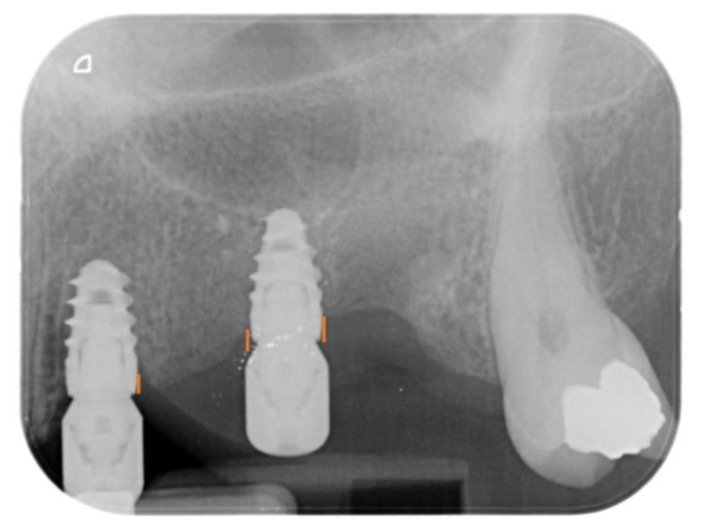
Periapical X-rays showing the distance between the implant-abutment interface and the most coronal aspect of the bone. Left implant: implant with machined abutment; Right implant: implant with superlattice abutment.
